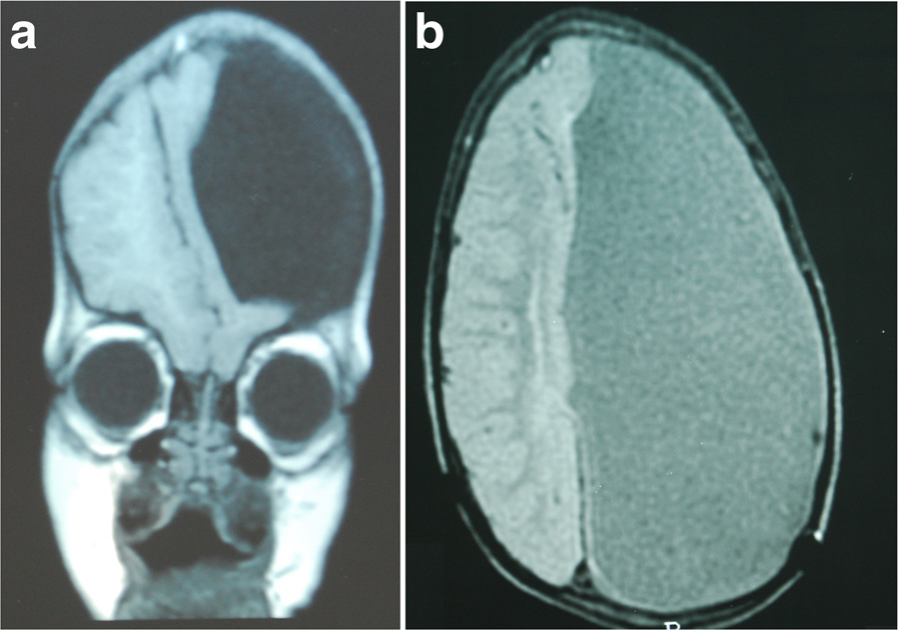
a: MRI sagittal T1 scan demonstrating a left-sided hemihydranencephaly with replacement of the left cerebral cortex with cerebrospinal fluid-filled sacs at the age of 1 year. b: MRI coronal T2 scan demonstrating left-sided hemihydranencephaly at the age of 2,5 year.
